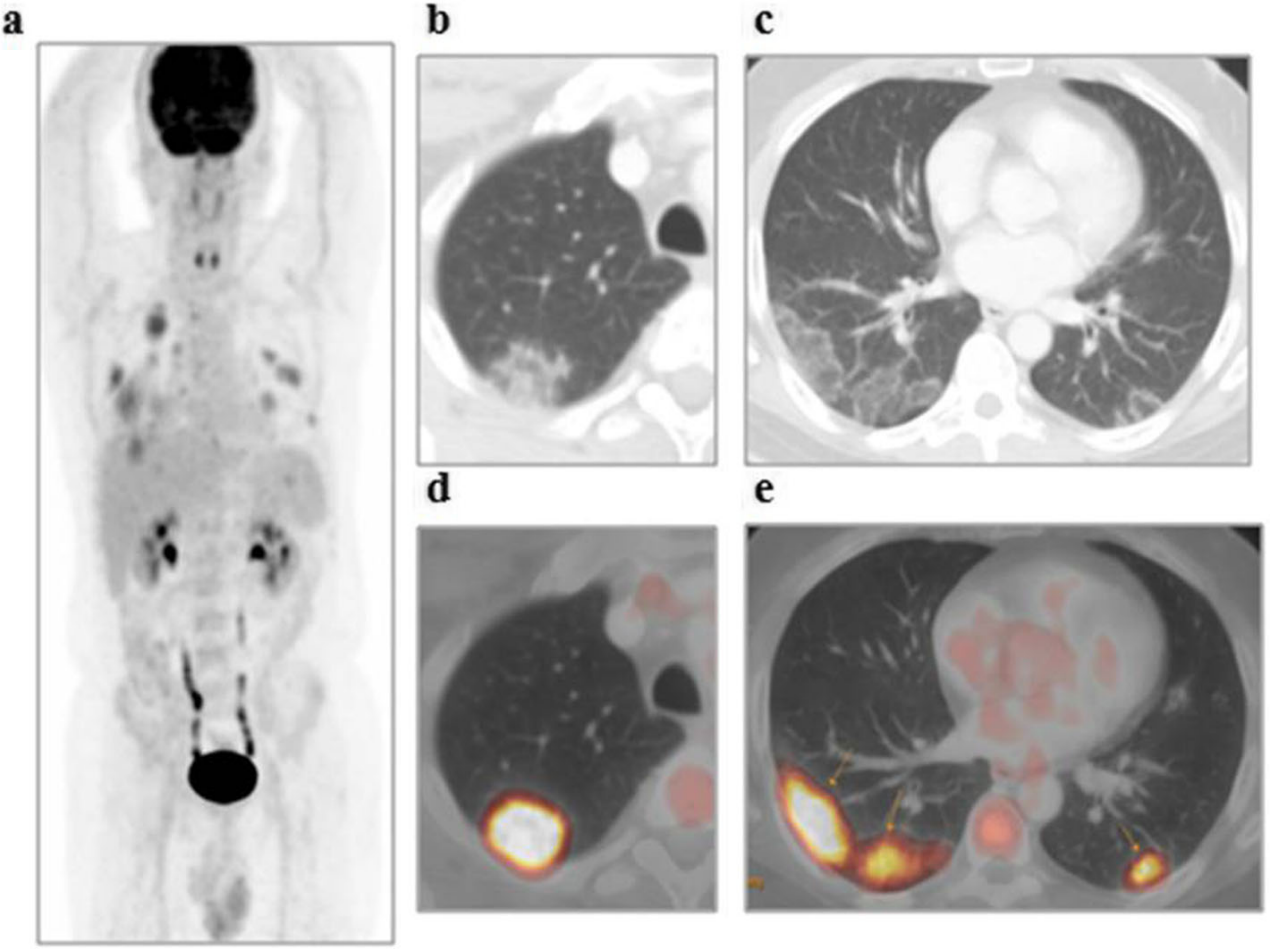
A 51-year-old male patient, with a history of treated lymphoma 5 years ago, referred for follow-up PET/CT evaluation. a The whole-body MIP image shows multiple bilateral pulmonary metabolically active areas. Axial lung window CT images (b, c) revealed multiple bilateral subpleural variable-sized ground-glass opacities associated with septal thickening (crazy-paving pattern) that show variable FDG avidity on corresponding fused PET/CT images (d, e) achieving up to 7.4 SUVmax
